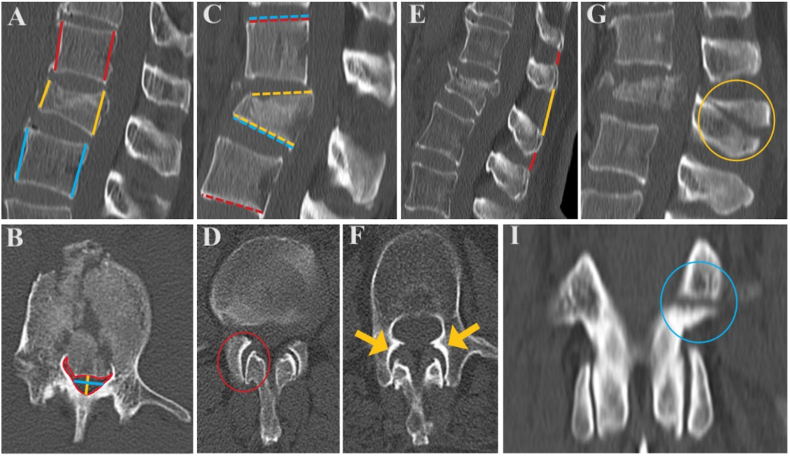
CT parameters used for diagnosis of PLC injury. CT sagittal images (A, C, E, G) showing (A) measurement of the UAVH and UPVH (red lines), AVH and PVH (yellow lines), LAVH and LPVH (blue lines); (C) measurement of LK (yellow dashed lines), RK (red dashed lines), GDA (blue dashed lines); (E) ISD >4 mm (yellow line) compared with adjacent levels (red lines); (G) a SPF (yellow circle). CT axial images (B, D, F) showing (B) measurement of the MSD (yellow line), TD (blue line) and direct measurement of TCA (red line); (D) FJW (red circle); (F) bilateral FJM (yellow arrows). CT coronal image (I) showing unilateral horizontal laminar fracture (blue circle). (For interpretation of the references to colour in this figure legend, the reader is referred to the Web version of this article.)
